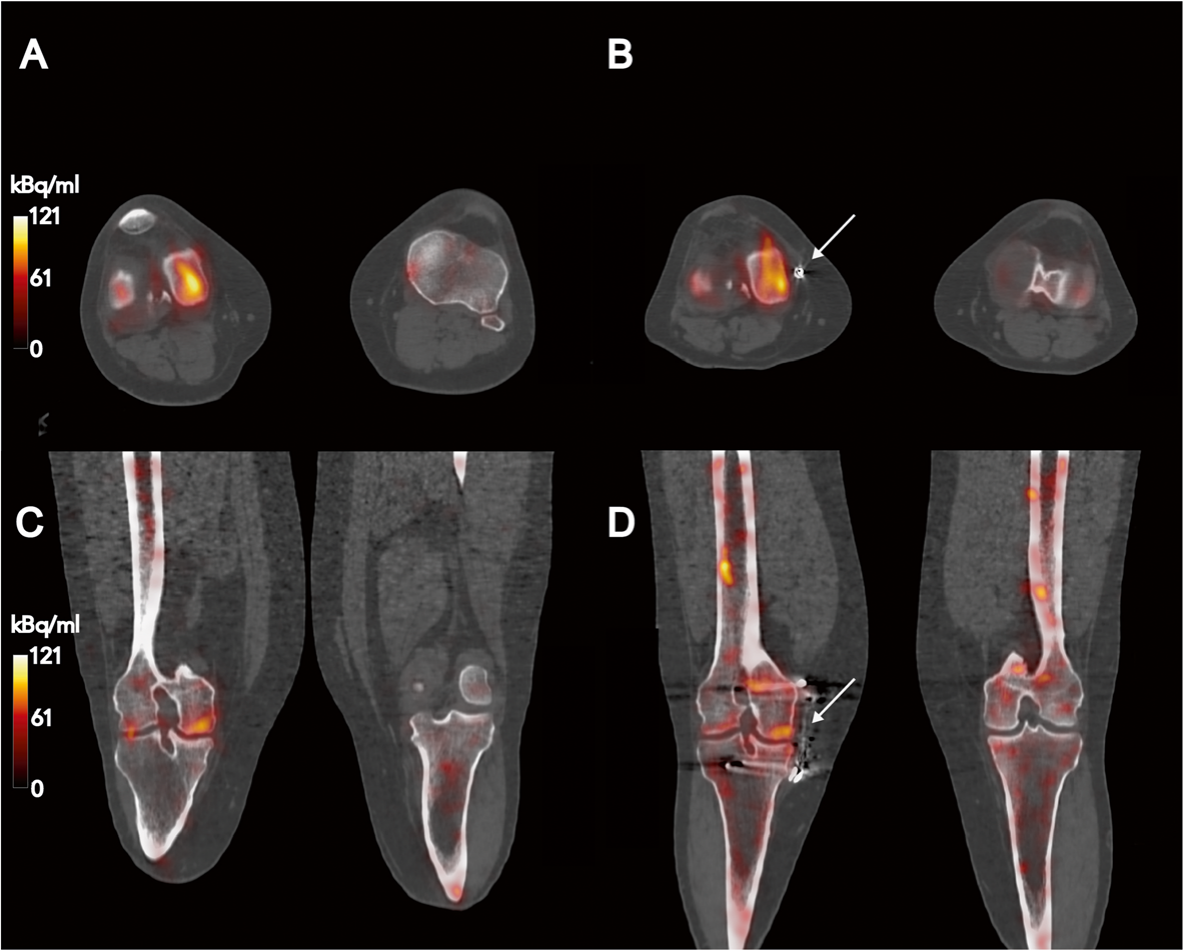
a, c Pre-op SPECT-CT images from a patient (f, 36 year, SPECT-CT: 8 months pre-implantation) with a secondary gonarthrosis and b, d corresponding post-op imaging (10 months post-implantation) exemplifying the effect from the examined knee implant system (b, d: metal artifact labeled by arrow). Patient showing a constantly increased uptake at the medial condyle of the right femur after implantation of the load absorber (AC_max, pre_ = 118.6 kBq/ml [SUV_max_ = 35.6] and AC_max, post_ = 110.89 kBq/ml, [SUV_max_ = 32.9]). Accumulation patterns from bone turnover (pre/post) were windowed identically
